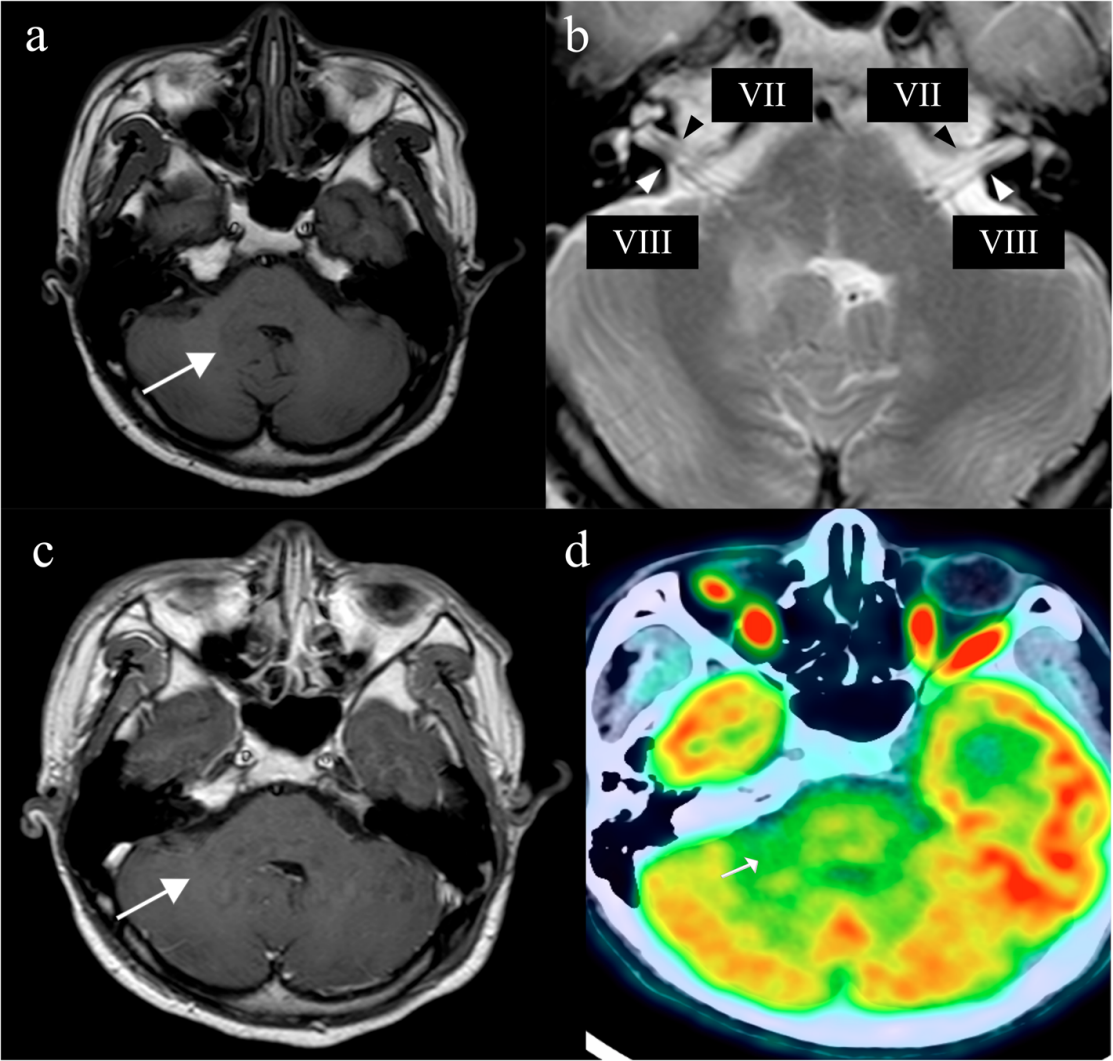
Axial T1 (a), T2 (b), and T1-weighted contrast-enhanced (c) MR images demonstrate an expansile ill-defined patchy lesion involving the right side of the middle cerebellar peduncle and dentate nucleus (*arrows*) as well as the displacement of the fourth ventricle. However, the ipsilateral facial nerve (*black arrowhead*) and vestibulocochlear nerve (*white arrowhead*) were not affected. The fused PET image (d) of the ^18^F-FDG PET/CT scan presents a decreasing metabolic lesion (SUVmax = 6.3) in the right brachium pontis that involves the ipsilateral cerebellar dentate nucleus (*arrows*)
